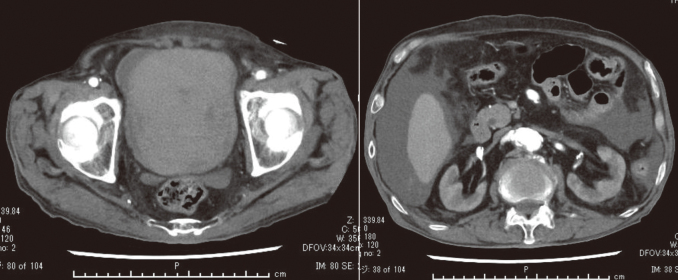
CT shows that the bladder was filled with hematoma on the left and the ascites was detected in the abdominal cavity on the right.
